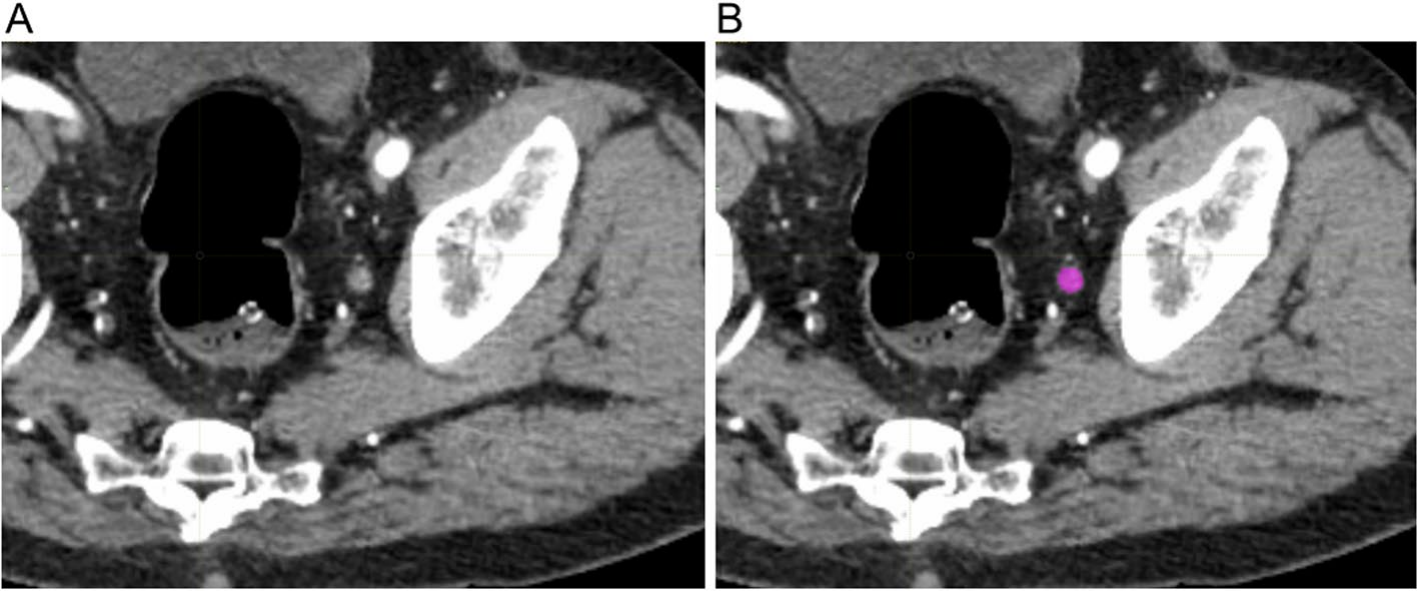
Analysis of the texture parameters on the axial first-phase computed tomography (CT) image that yielded the maximum lateral pelvic lymph node area. A An enlarged lateral pelvic lymph node. B The region of interest was drawn manually along the outer edge of the lymph node and the texture parameters were extracted
